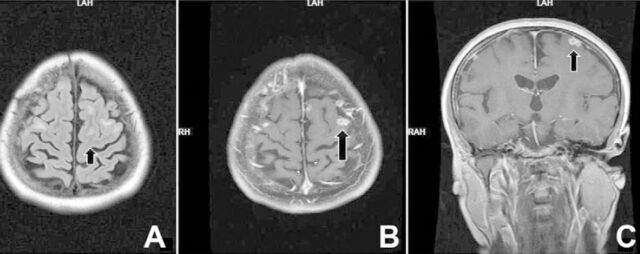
Patient MRI showing A) Axial Flair, B) postcontrast T1 weighted axial, and C) coronal images acquired at the same plane as figure 1 after 6 months of treatment. There is a decrease in size and number of tuberculomas, together with decreasing edema.
